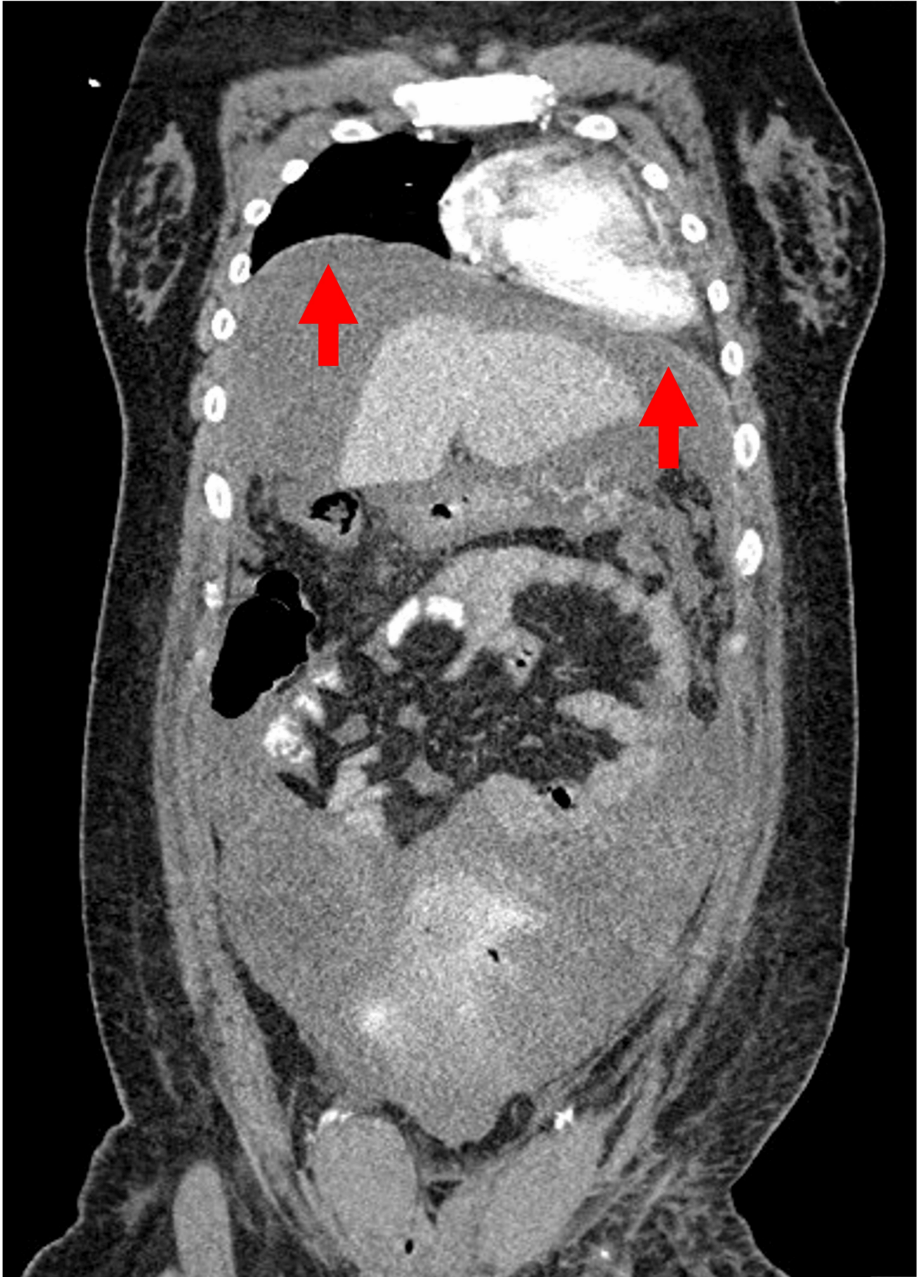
CT image at cardiopulmonary arrest. The dome of the diaphragm was elevated with the 5th thoracic vertebral body.
